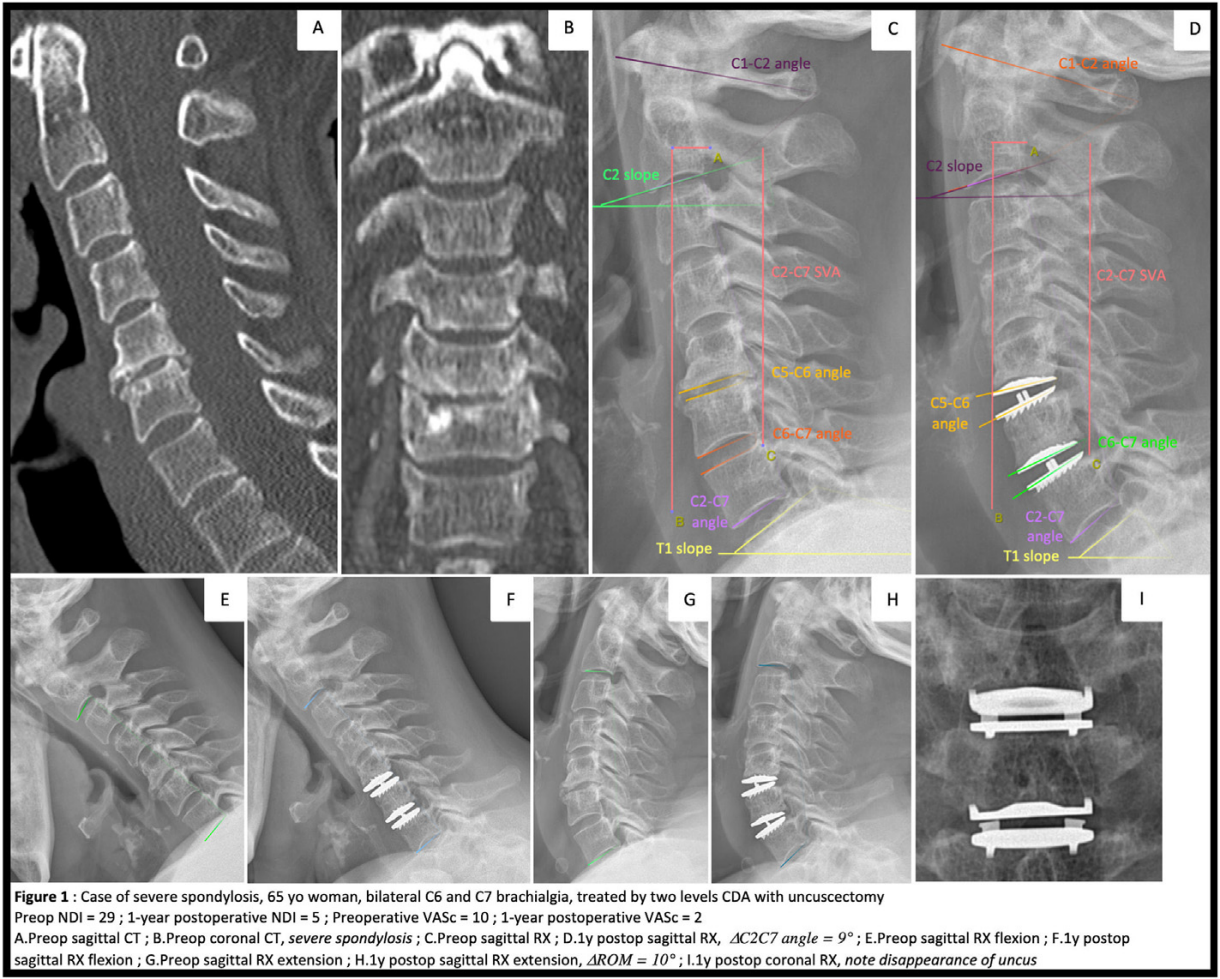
Case of severe spondylosis, 65 yo woman, bilateral C6 and C7 brachialgia, treated by two levels CDA with uncuscectomy Preop NDI ​= ​29; 1-year postoperative NDI ​= ​5; Preoperative VASc ​= ​10; 1-year postoperative VASc ​= ​2 A. Preop sagittal CT; B. Preop coronal CT, severe spondylosis; C. Preop sagittal RX; D.1 ​y postop sagittal RX, *ΔC2C7 angle* ​= ​*9°*; E. Preop sagittal RX flexion; F.1 ​y postop sagittal RX flexion; G. Preop sagittal RX extension; H.1 ​y postop sagittal RX extension, *ΔROM* ​= ​*10°*; 1 ​y postop coronal RX, note disappearance of uncus.
